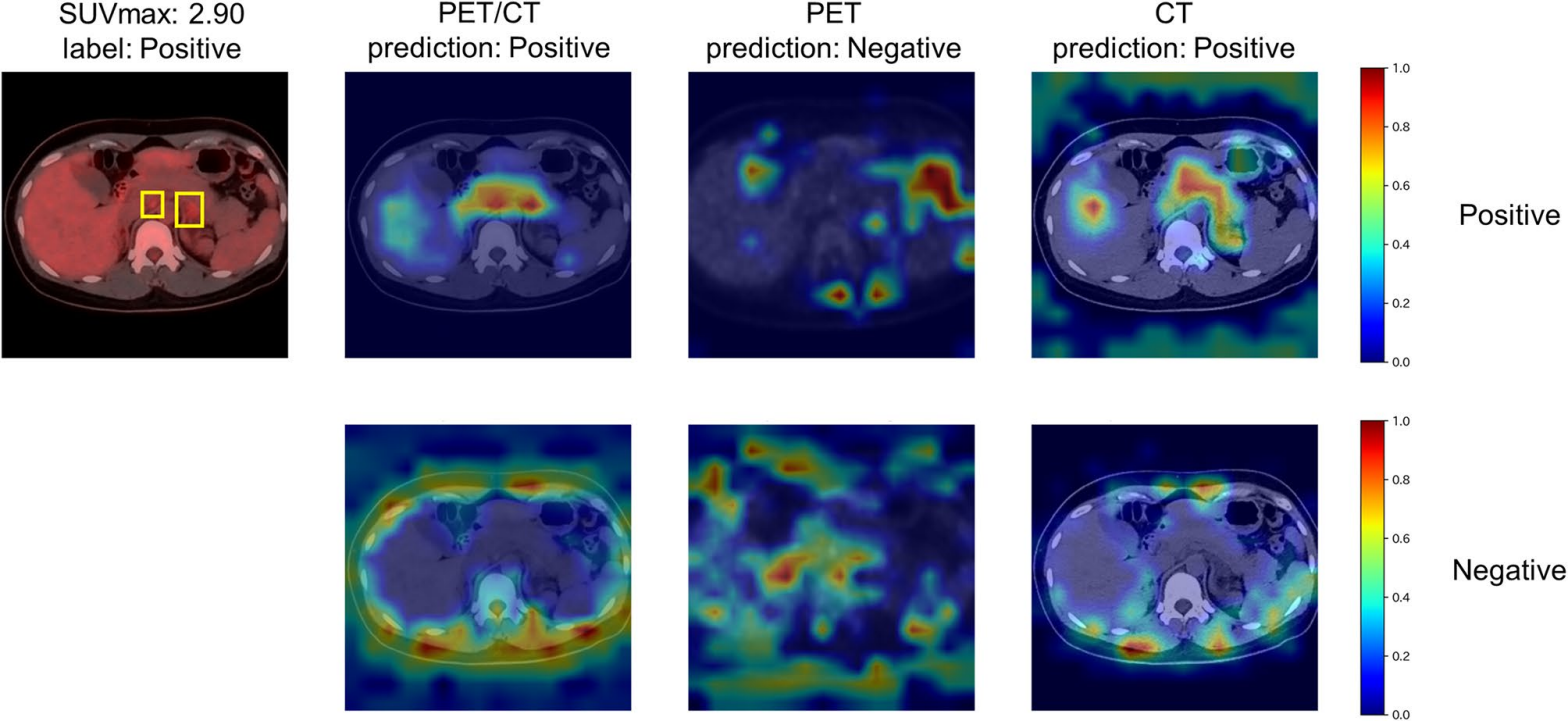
Predictions and Grad-CAMs of ViT-based models on sample PET/CT, PET, and CT test images from the “positive” class. ViT was fine-tuned using training data of each modality. The bounding boxes depicted in the figure indicate malignant lesions. The top row of the Grad-CAMs shows important areas for “positive” predictions, and the bottom row shows areas for “negative” predictions. *PET* Positron emission tomography, *CT* Computed tomography, *SUVmax* Maximum standardized uptake value, *ViT* Vision Transformer, *Grad-CAM* Gradient-weighted class activation mapping.
